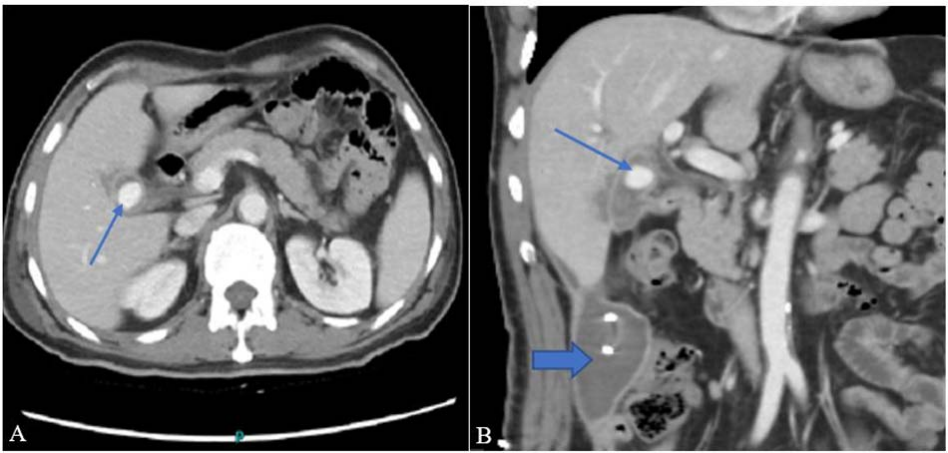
(A) Contrast-enhanced CT axial image through the abdomen shows a small walled-off collection in the GB fossa with a central contrast density representing pseudoaneurysm (arrow). The collection appears to be in communication with the second part of the duodenum. (B) A coronal image from the same CT shows a right para colic gutter collection with an *in situ* pigtail (broad arrow).
